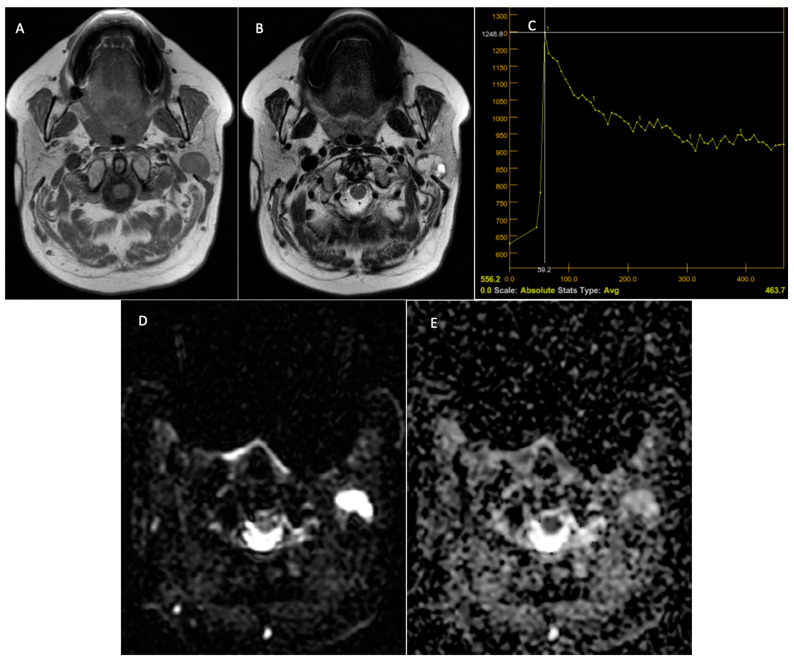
Warthin tumor—left parotid tumor. Preoperative MRI. T1-weighted sequence with a hypointense signal at the level of the tumor, a well-demarcated lesion (A). T2 sequence—mixed signal at the level of the lesion and the presence of cystic areas at the level of the lesion (B). DCE-MRI—C-type TIC curve, with rapid contrast uptake and rapid washout (C). The DWI-MRI sequences (D) demonstrate intratumoral diffusion restriction, with the ADC map (E) having a value of 0.73 × 10^−3^ mm^2^/s.
